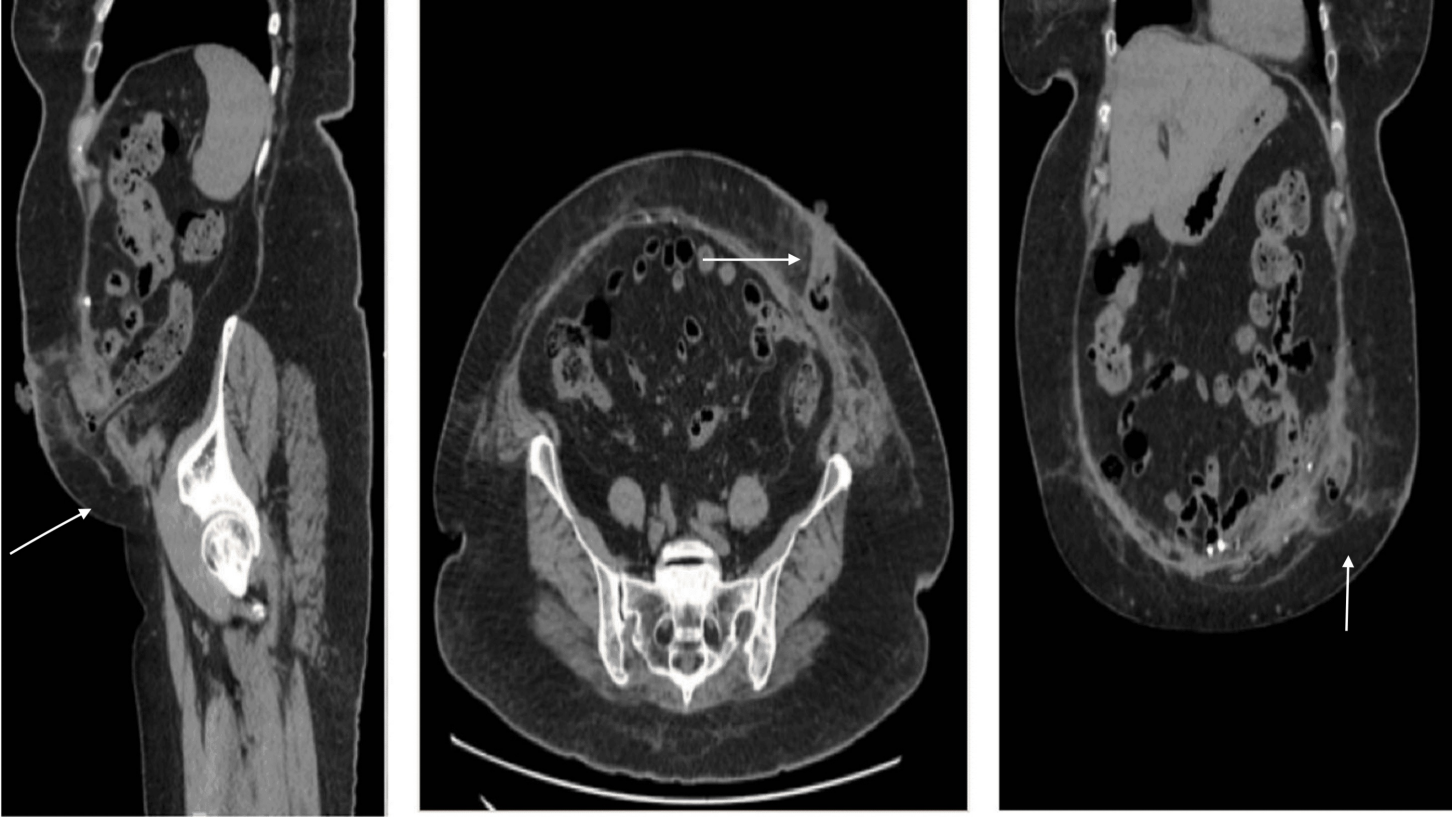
Postoperative CT scan confirming intact onlay mesh repair and absence of hernia recurrence at six weeks postoperatively.
